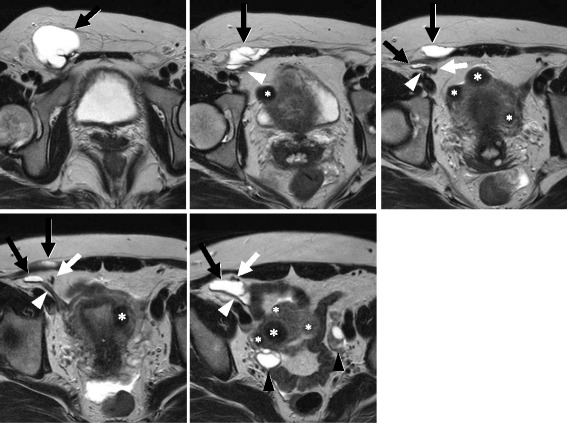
Axial T2-weighted MRI. Axial T2-weighted MRI shows a cystic mass (*black arrows*) measuring 48 × 37 mm in the right inguinal region. A smaller cystic lesion and fluid collection extend along the right round ligament in the pelvic cavity. Round ligament (*white arrowheads*), inferior epigastric artery and vein (*white arrows*), bilateral ovary (*black arrowheads*), and myomas (*asterisks*)
